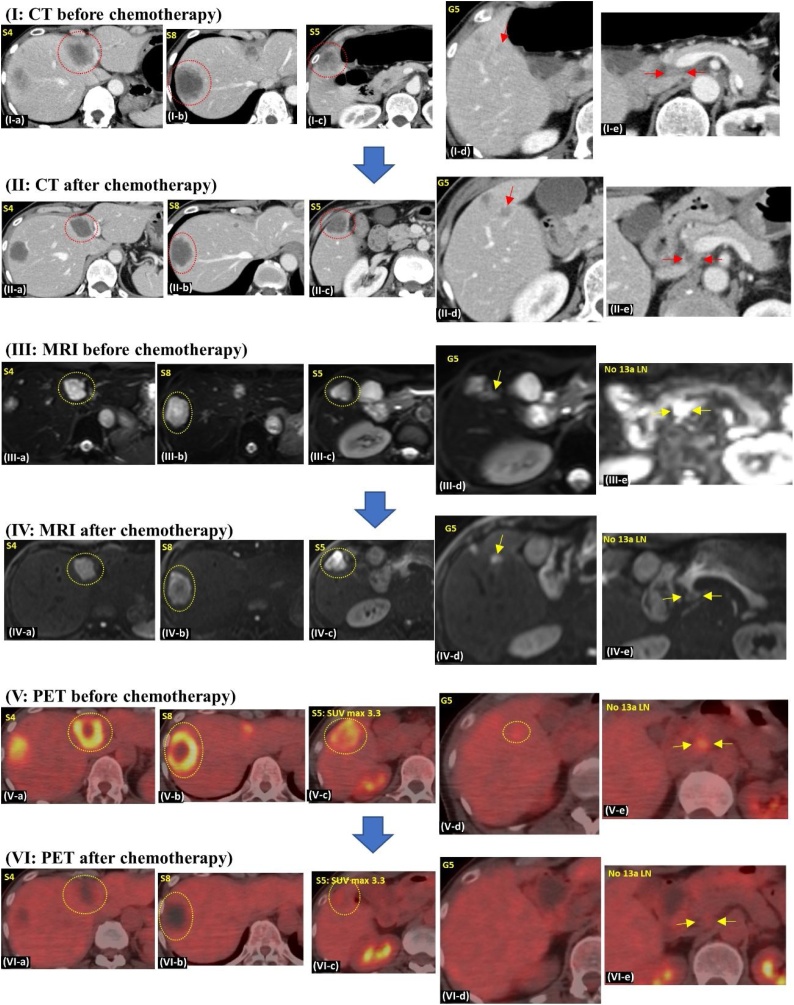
I-a, b, c) Three metastatic lesions noted in the liver (S4: size 44 mm, S8: size 45 mm, and S5: size 35 mm). I-d) Suspected tumor progression along Glisson 5 (red arrow). I-e) A retropancreatic LN of size 12 mm is seen (red arrow). II-a, b, c) All liver metastatic lesions reduced in size (S4: size 30 mm, S8: size 40 mm, S5: size 28 mm). II-d) Tumor progression along Glisson 5 is more evident (red arrow). II-e) Retropancreatic LN have reduced in size (10 mm; red arrow). III-a, b, c) DWI-MRI revealing high intensity areas concomitant with the liver metastases. III-d) DWI-MRI showing high intensity signal along Glisson 5. III-e) DWI of MRI showing high intensity signals in the retropancreatic LN. IV-a, b, c, d, e) DWI-MRI revealing high intensity signals consistent with suspected metastatic lesions. V-a, b, c, d, e) PET revealing high intensity signals, all consistent with suspected metastatic lesions including retro-pancreatic LN. VI-a, b, c) Although S5 metastasis still shows a weak intensity signal, there is no detectable signal intensity in the S4 and S8 metastases. VI-d, e) PET does not show any signal intensity in Glisson 5 and the retropancreatic LN.
